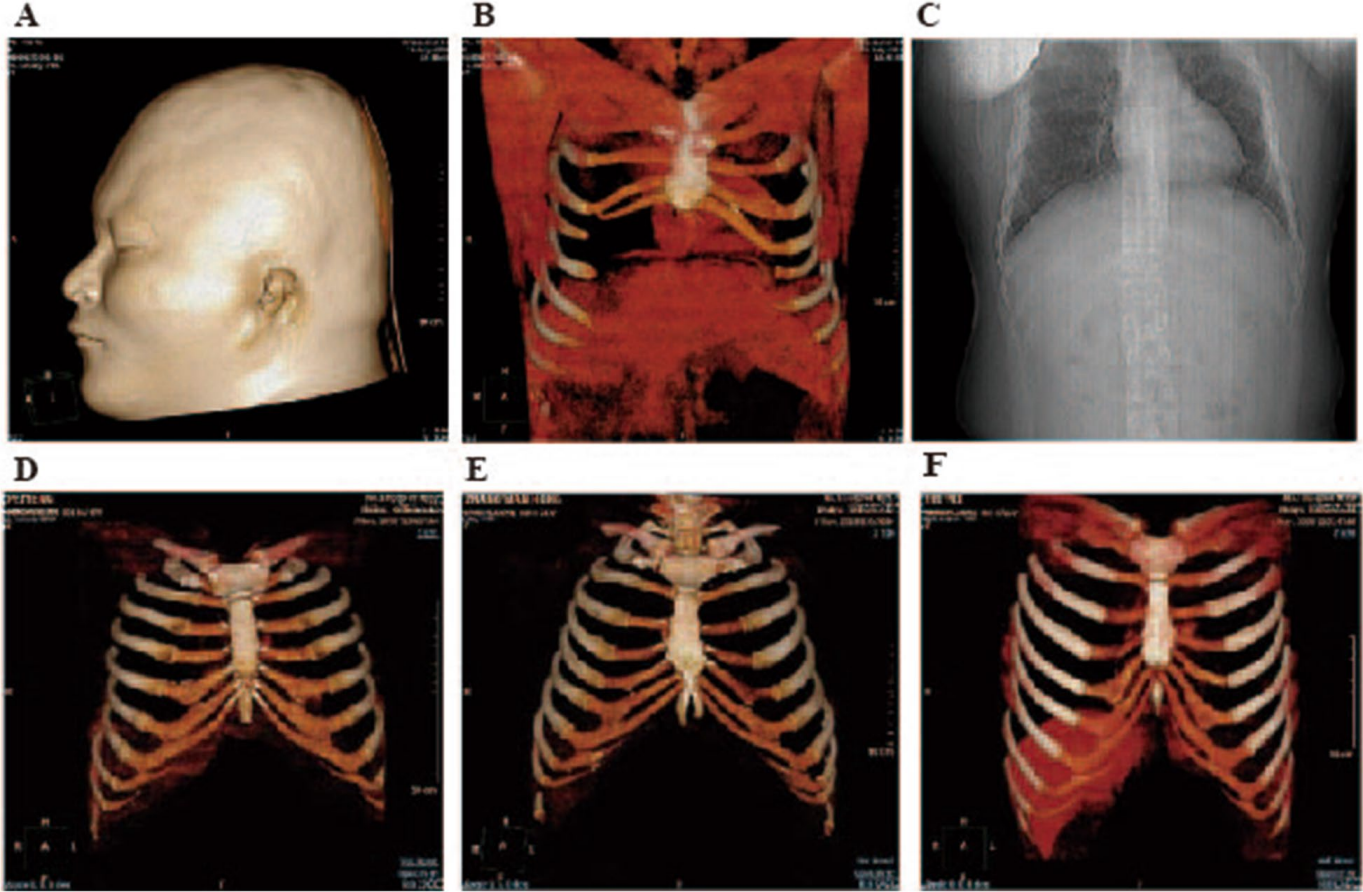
The phenotype of the patients and family members A Three-dimensional (3D) Computed Tomography (CT) reconstructions of the patient head showed left-side microtia B 3D-CT reconstruction of the patient costal area showed costal chondrodysplasia with sternal dysplasia. The costal cartilage is missing on the 6–10 and not attached to the rib. C X-ray thorax shows no deformity of the vertebral column (D-F) 3D-CT results of the patient's father (D), mother E and sister’s F normal costal area
